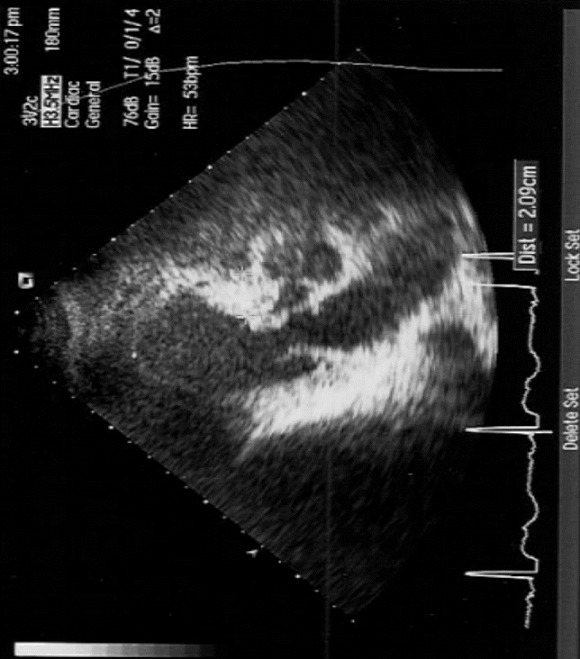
Transthoracic ultrasonography showed an asymmetrically overdeveloped left ventricle
